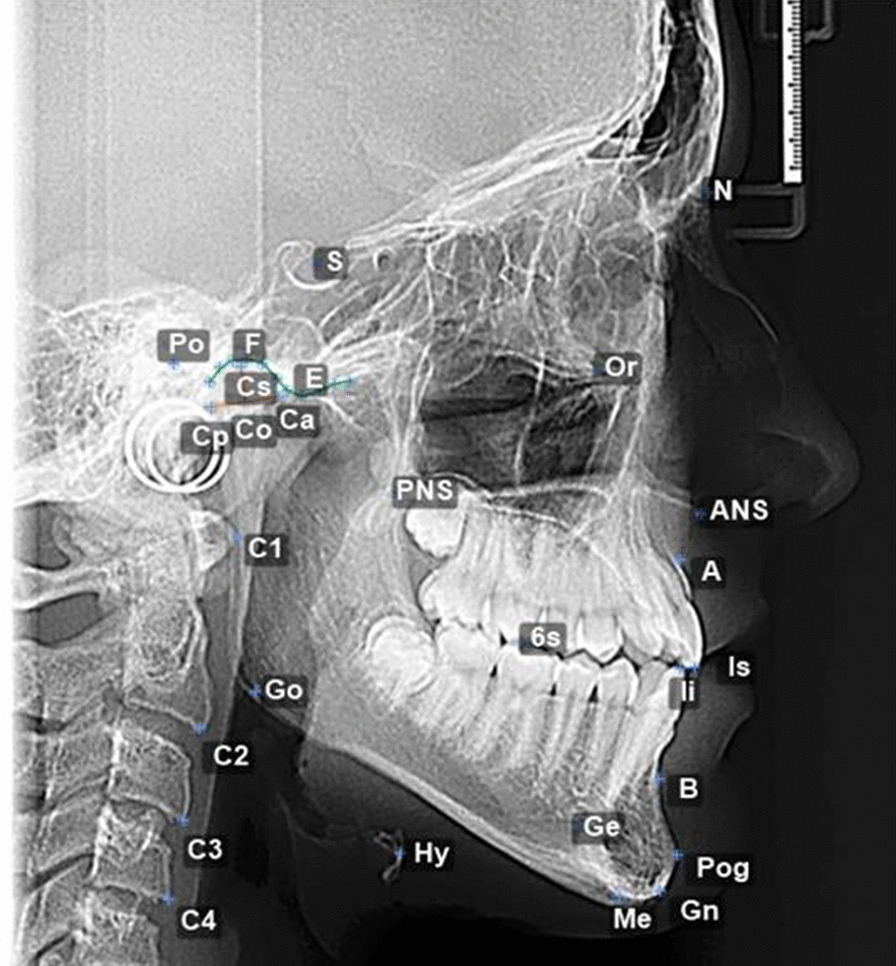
Cephalometric landmarks. Shows cephalometric radiography with landmarks as described in Table 1
